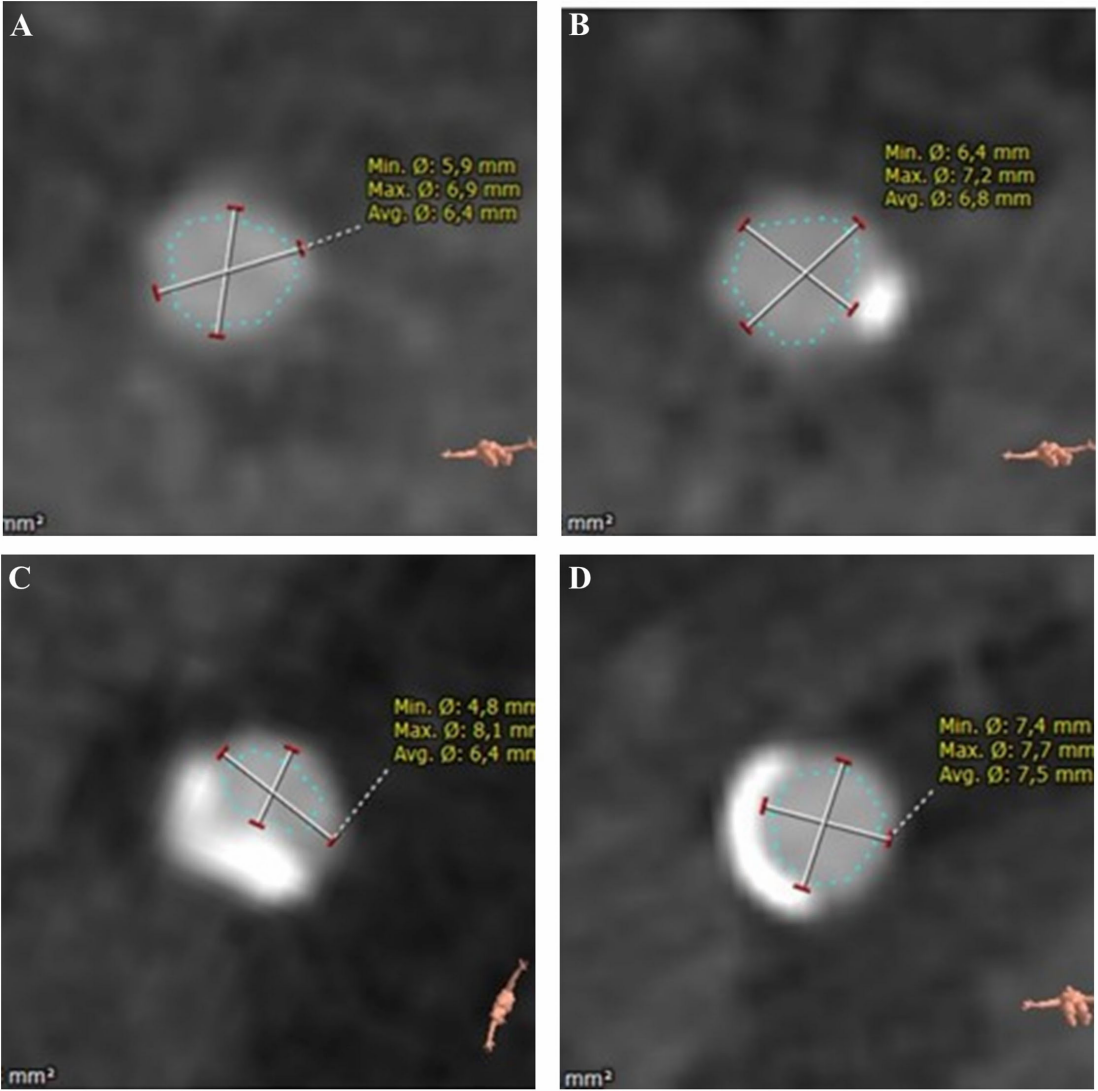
Quantification of calcification of common femoral artery (derived from CT): none (A) mild (B) severe (C,D).
